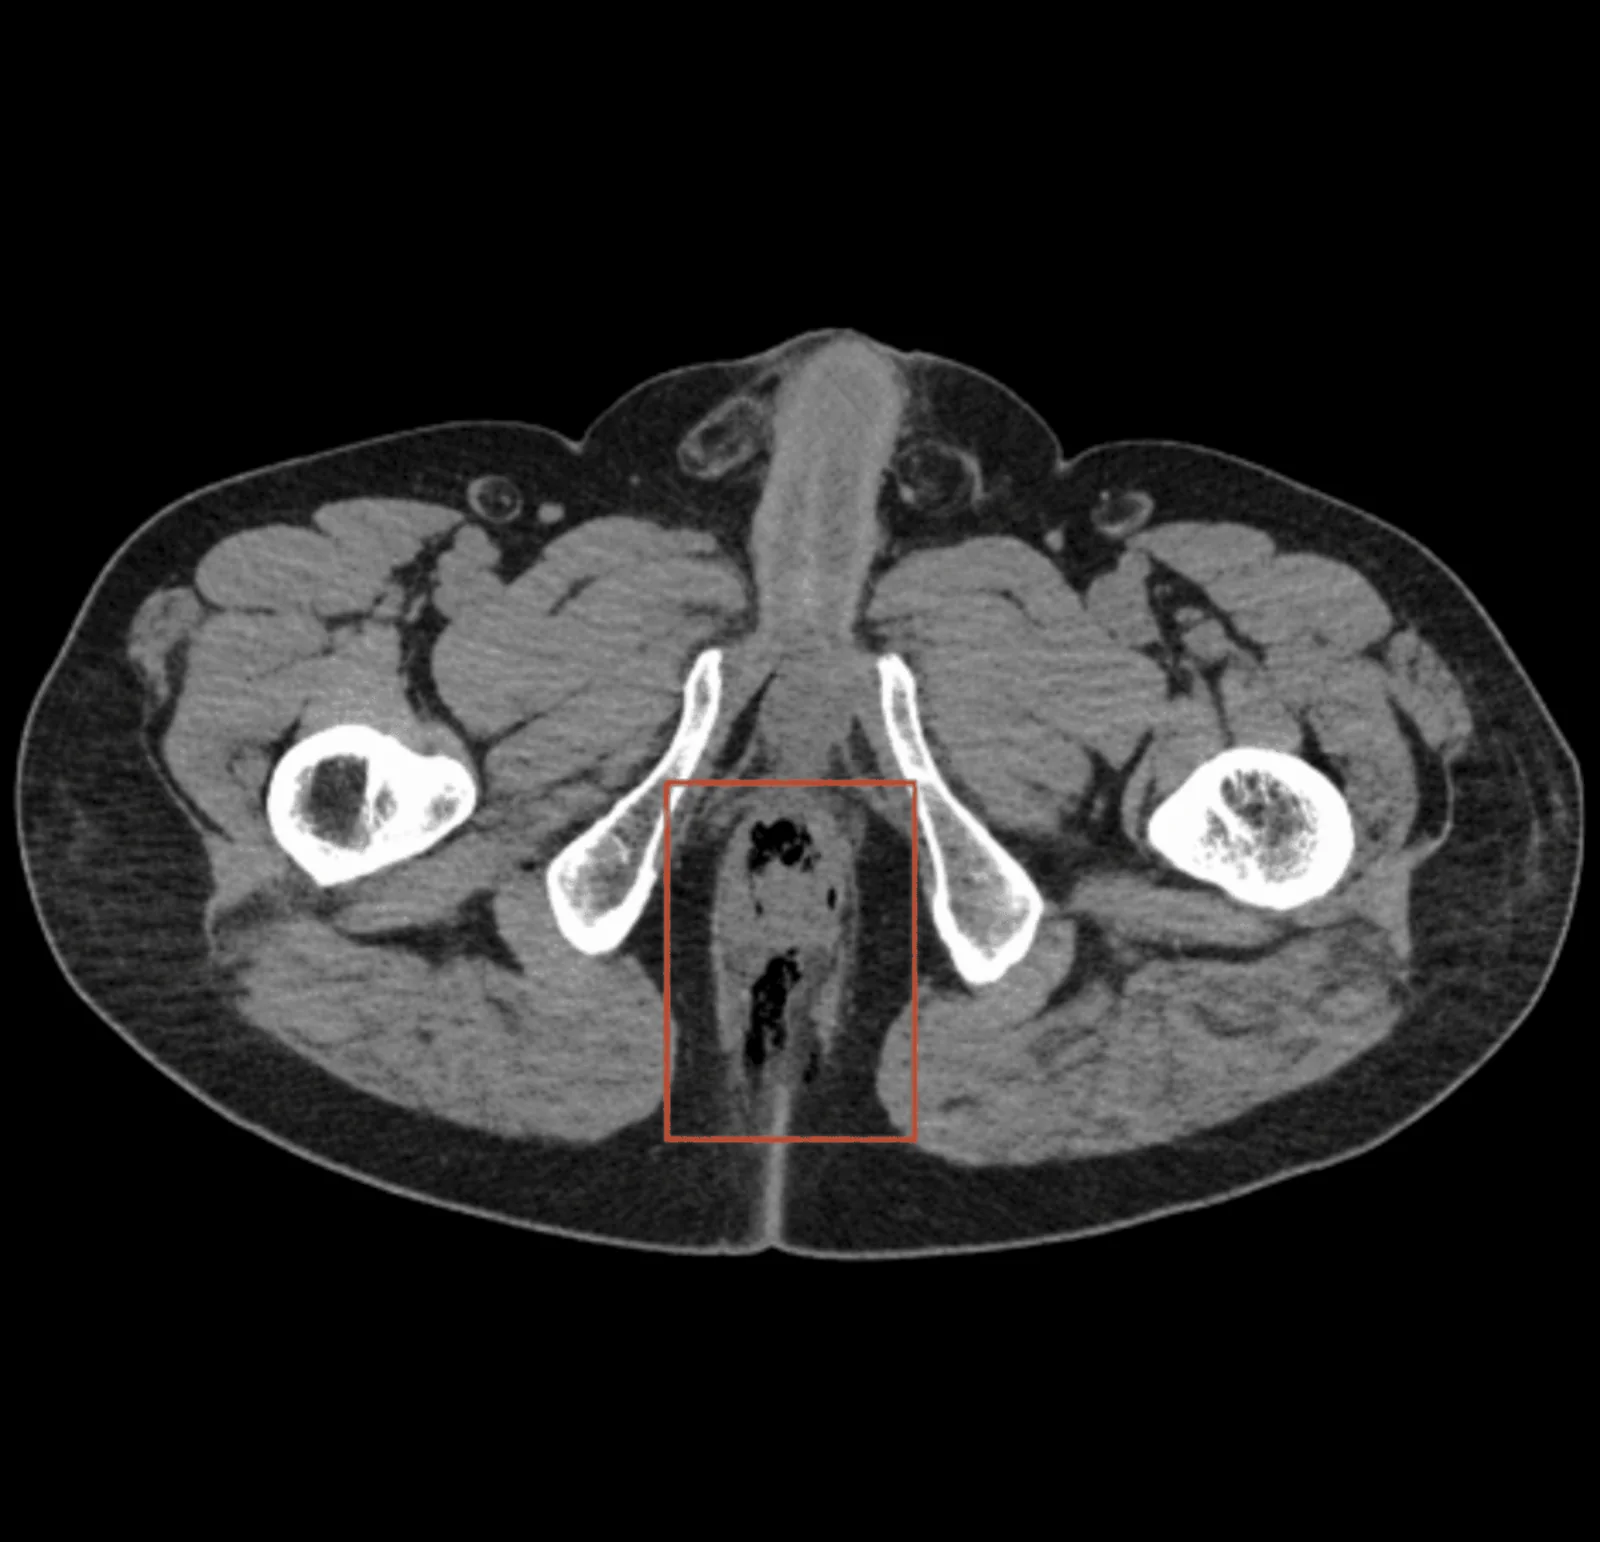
Axial computed tomography of the abdomen and pelvis. Axial computed tomography (CT) image of the abdomen and pelvis showing soft tissue gas surrounding the anorectal junction and extending into the perineum (red box), concerning for Fournier gangrene.
